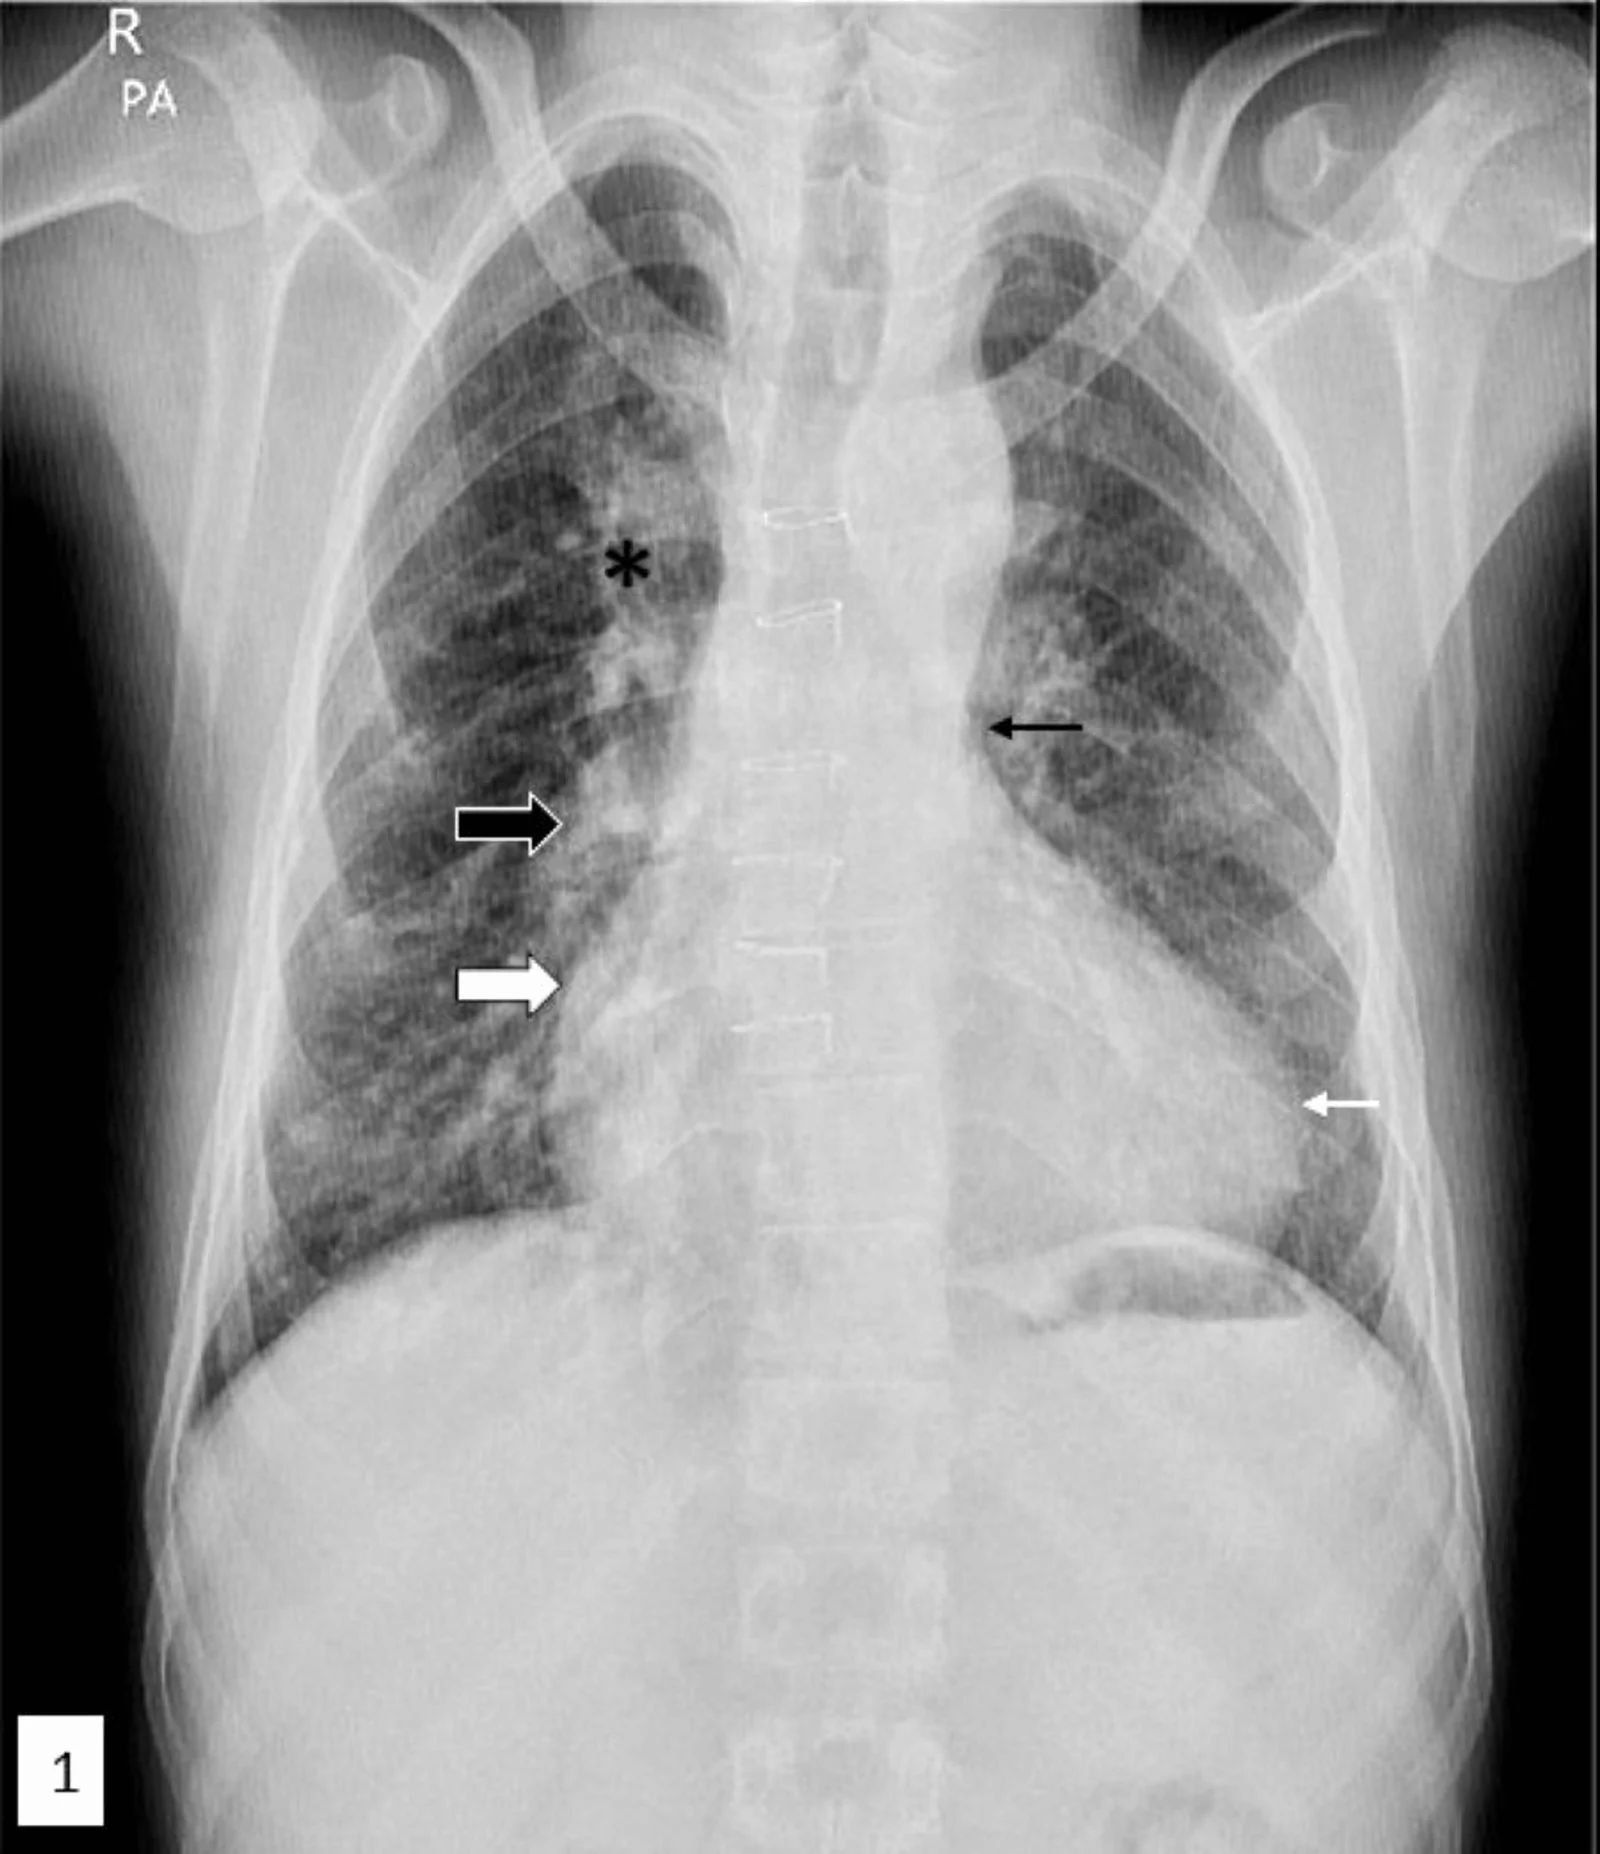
Chest radiograph of the patient Posteroanterior chest radiograph showing mild cardiomegaly with prominence of the right cardiac border (white block arrow) and slightly upturned cardiac apex (white arrow). There is marked concavity at the level of the pulmonary artery segment along the left heart border with loss of the normal 2nd mogul (black arrow). There is increased lung markings in the right lung (asterisk) with a prominent right descending pulmonary artery (black block arrow).
